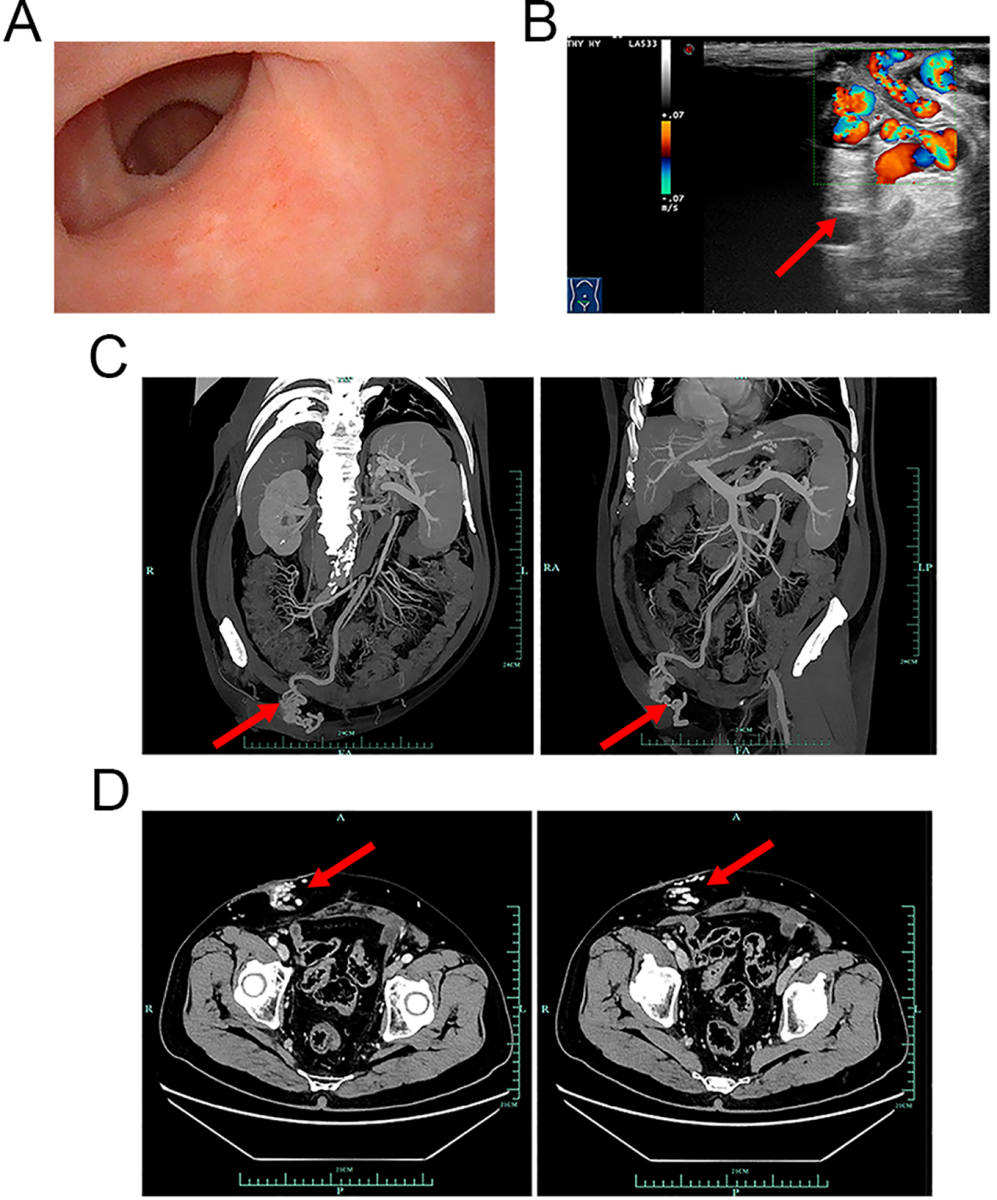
Cystoscopy, ultrasonic, and CTA examination images. (A) Cystoscopy revealed that the mucosa of the ileal conduit was smooth. No obvious varicose veins, bleeding points, or tumour recurrence was observed. (B) CFDI showing that the vessels around the vesicostomy are tortuous, extending upwards to the mesenteric vessels and downwards to the iliac veins. Local luminal blood flow signals were abundant, with a flow velocity of 20 cm/s (red arrow). (C, D) CTA images of the mesenteric artery showing that the varicose veins around the stoma were connected to the subcutaneous veins of the abdominal wall (red arrows). (C) Coronal and sagittal planes. (D) Cross-section. CTA, computed tomography angiography; CFDI, colour flow Doppler imaging.
